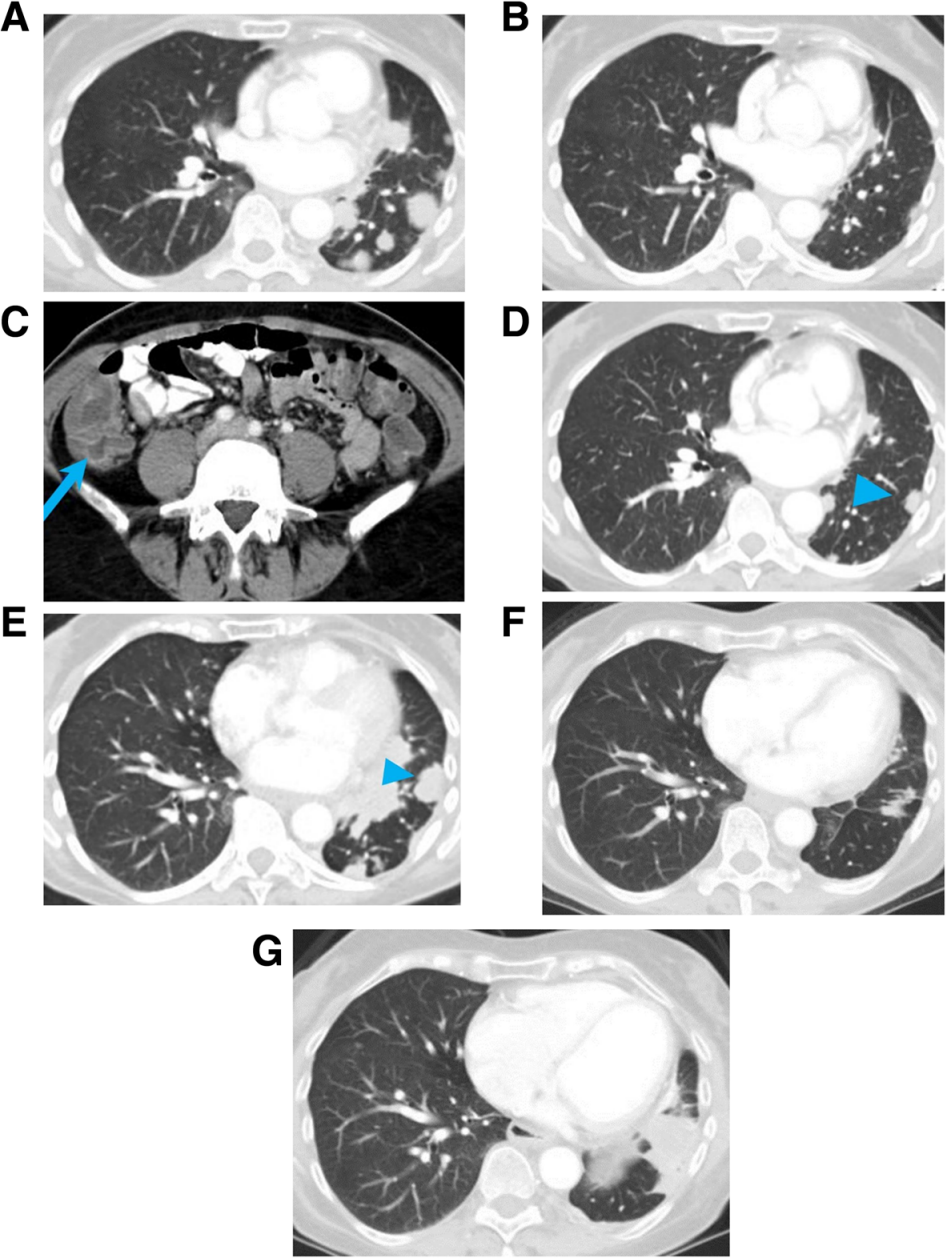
A 68-year-old nonsmoker woman with non-small cell lung cancer, with *EGFR* exon 19 deletion. Baseline CT of the chest (a) shows multiple lung nodules, which shrunk after 3 months of treatment with the EGFR inhibitor erlotinib (b). While on treatment, patient developed diarrhea, and CT of the abdomen acquired during portal venous phase showed fluid filled large bowel, consistent with drug induced colitis (arrow) (c). After 4 years of treatment, CT of the chest (d) showed a new left lower lobe nodule, which markedly increased in size in 3 months (arrowhead) (e). Biopsy of the nodule showed acquired T90M mutation, which confers resistance to erlotinib. Patient was switched to osimertinib, with initial improvement of the lung nodules on follow-up chest CT (f). Tumor burden remained stable for 2 years, until a chest CT scan showed increased right lower lobe nodule (g). Patient was then switched to pemetrexed
